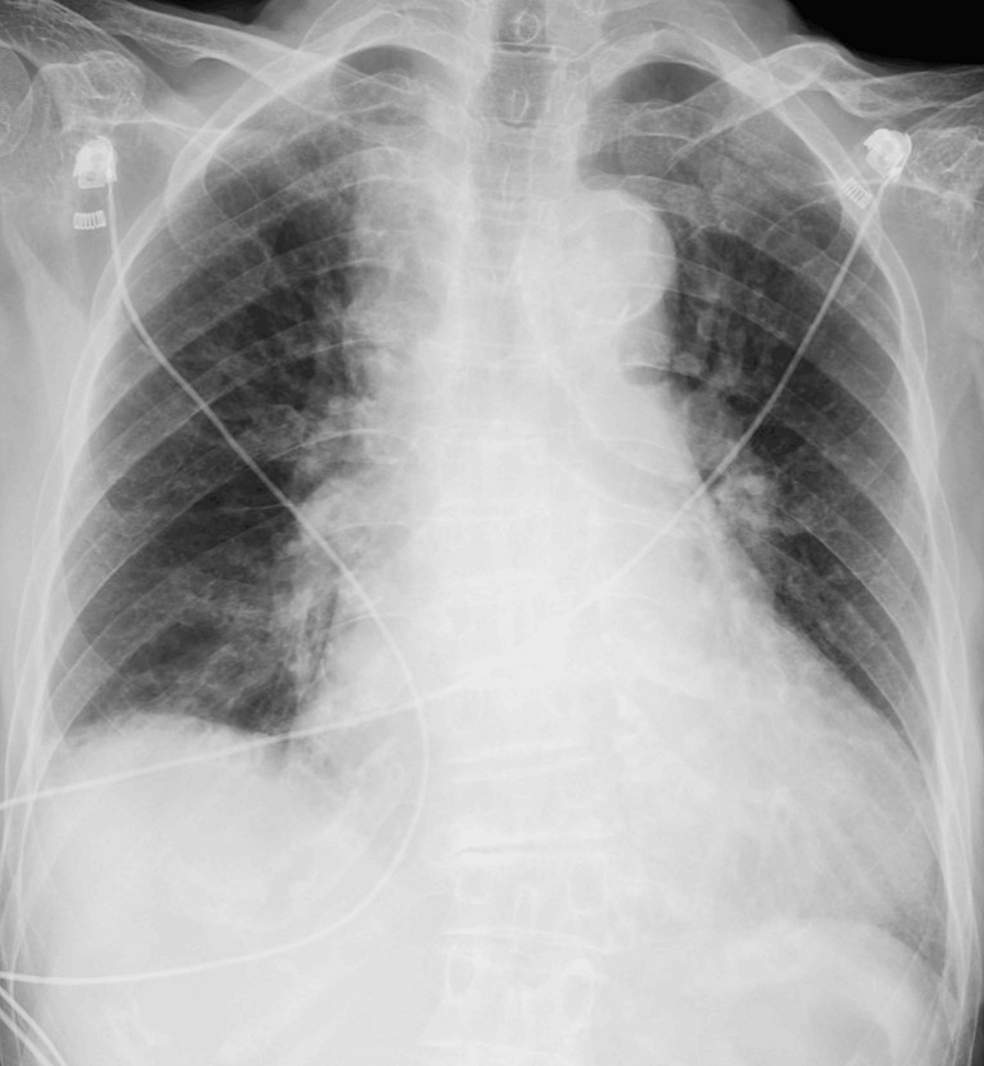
Chest radiograph revealed cardiomegaly and pulmonary edema.
